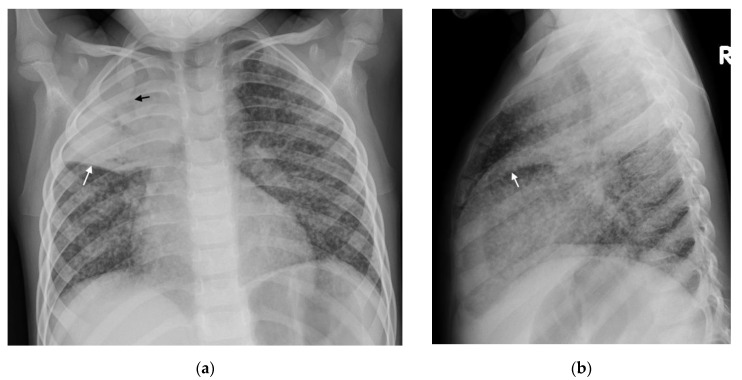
Consolidation and miliary TB. Frontal AP and lateral chest radiographs in a 3-year-old boy with confirmed disseminated TB. This child did not receive BCG vaccination. (a) Frontal AP and (b) lateral chest radiographs demonstrating right upper lobe consolidation with air bronchograms (black arrow) limited by the horizontal fissure (white arrows), as well as parenchymal miliary TB nodules.
